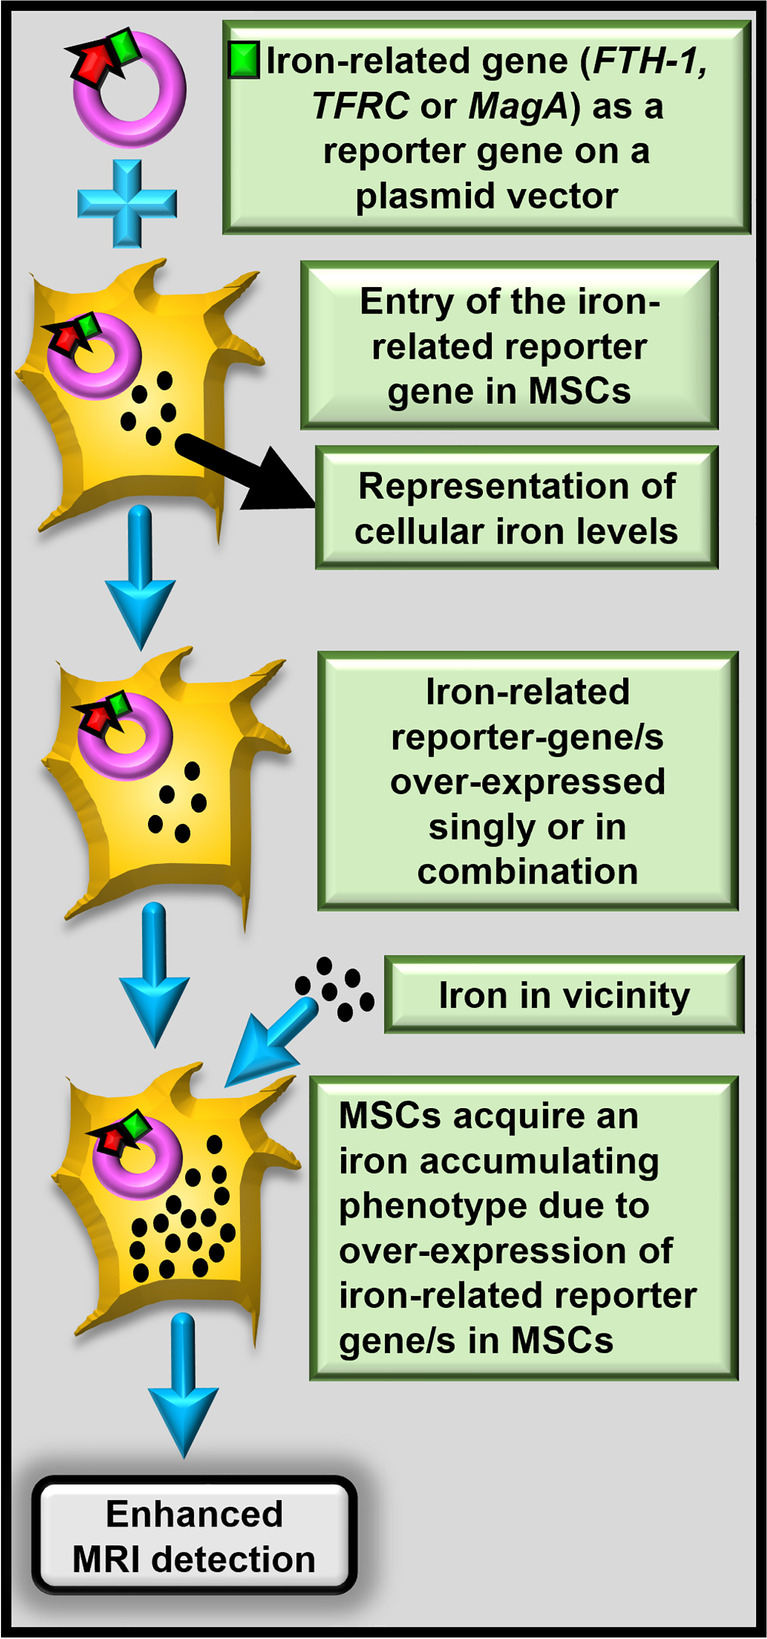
Principle of using iron-related reporter genes for MSC detection and tracking. The figure encapsulates the principle underlining the utilisation of iron-related genes (*FTH-1, TFRC* or *MagA*) as reporter genes in the MSCs to increase cellular iron accumulation and thereby improve cell detection via MRI
